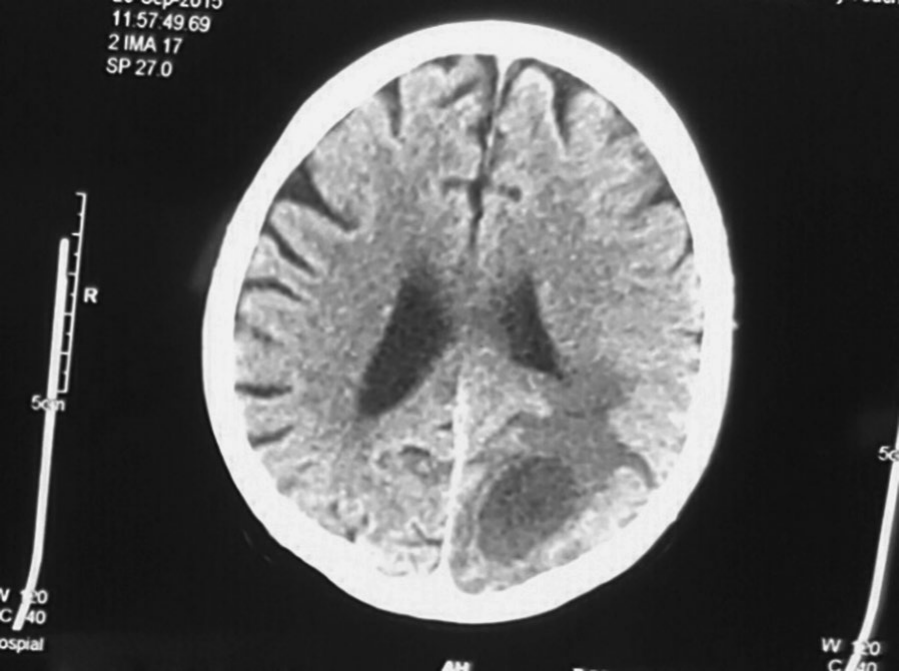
Head Computed tomography scan: ill-defined lesion with surrounding edema seen in *left* occipitoparietal region
